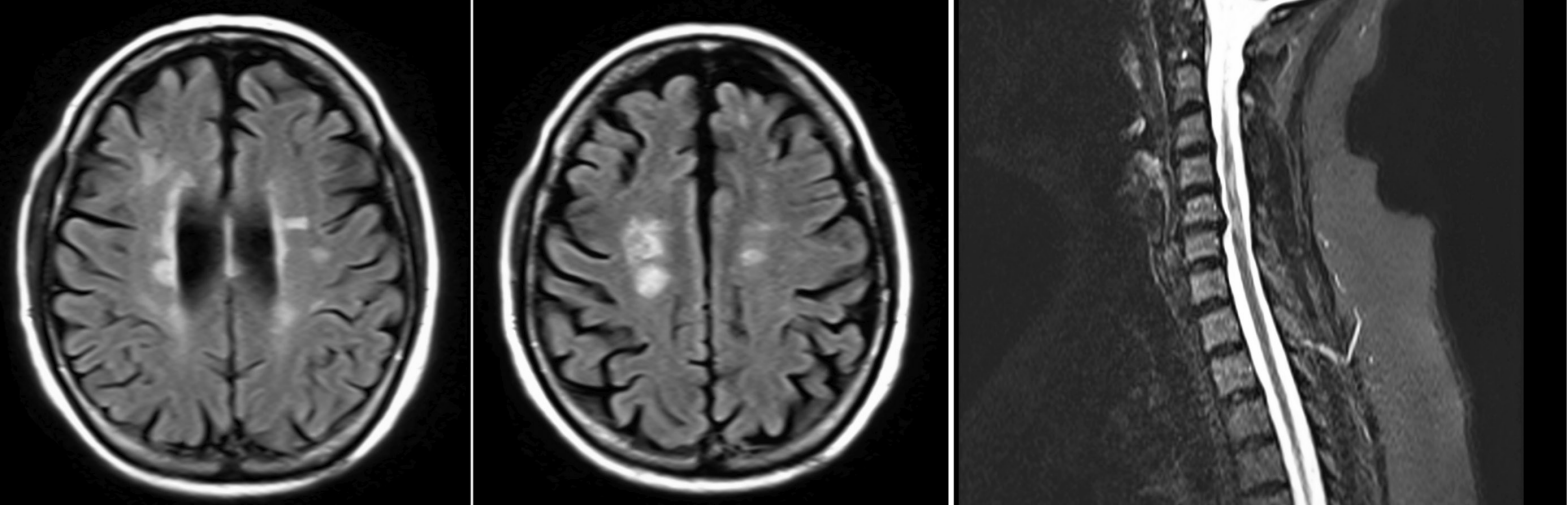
Case 1: fluid‐attenuated inversion recovery (FLAIR) MRI sequences showing high‐intensity areas in paraventricular and para‐spinal regions.
